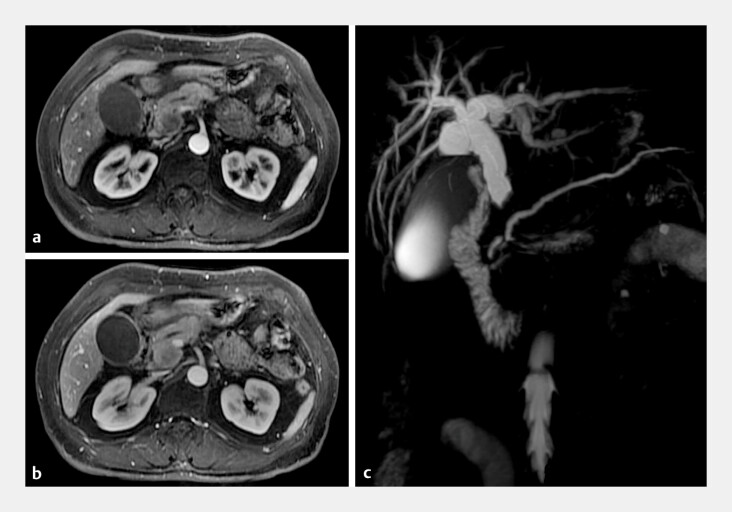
Enhanced magnetic resonance imaging and magnetic resonance cholangiopancreatography (MRCP) images showing:
